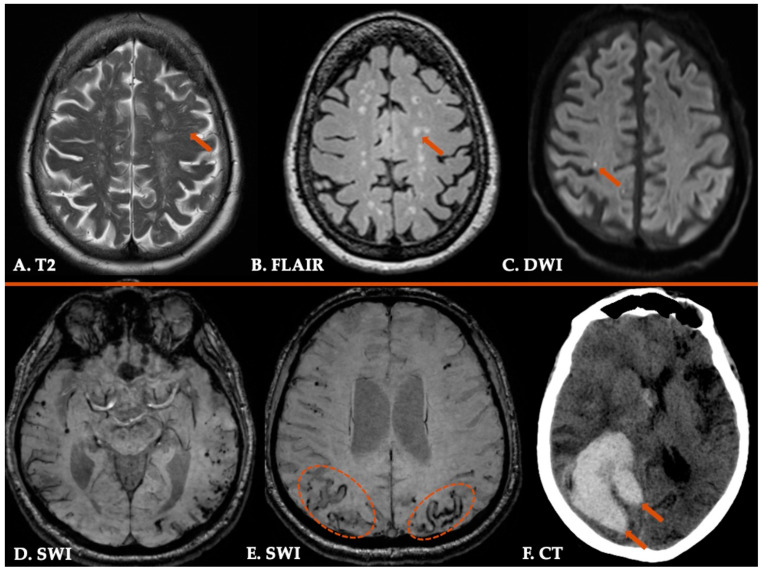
Neuroimaging findings in a patient with Cerebral Amyloid Angiopathy. Enlarged perivascular spaces in centrum semiovale (Panel (A)) and characteristic multispot white-matter hyperintensities pattern (Panel (B)) are detected in T2-weighted image and 3D fluid-attenuated inversion recovery sequence (FLAIR), respectively. Diffusion-weighted imaging (DWI) sequence (Panel (C)) showing a right parietal cortical microinfarct. Susceptibility-weighted imaging (SWI) sequences demonstrating multiple cerebral lobar microbleeds (Panel (D)) and disseminated cortical superficial siderosis (Panel (E)) in parietal lobes bilaterally. Fourteen months following CAA diagnosis, the patient presented focal neurological deficits attributed to a lobar hemorrhage of the right temporal lobe with characteristic finger-like projections (Panel (F)).
